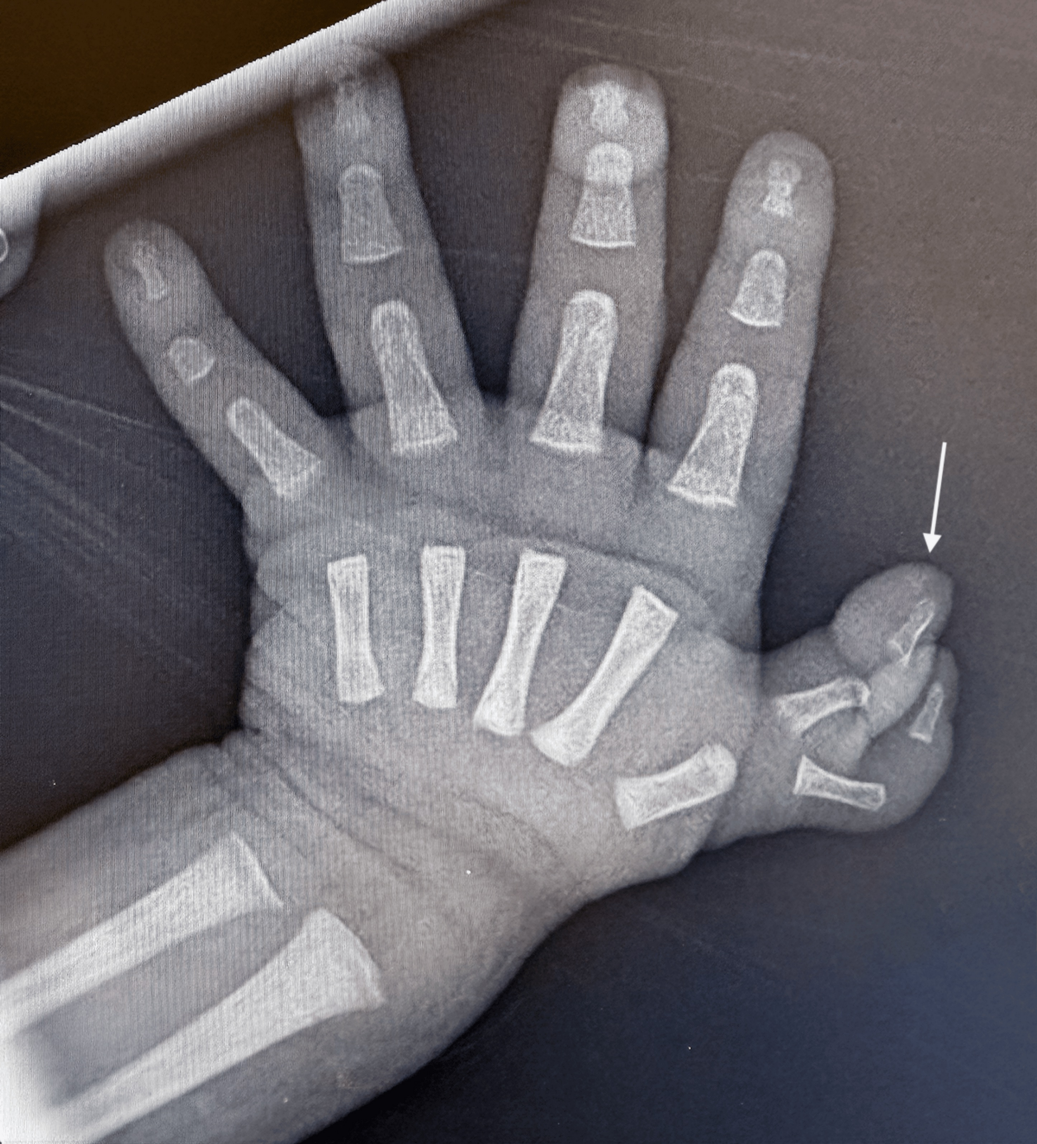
Anteroposterior radiograph of the right hand demonstrating a bifid thumb (white arrow)
